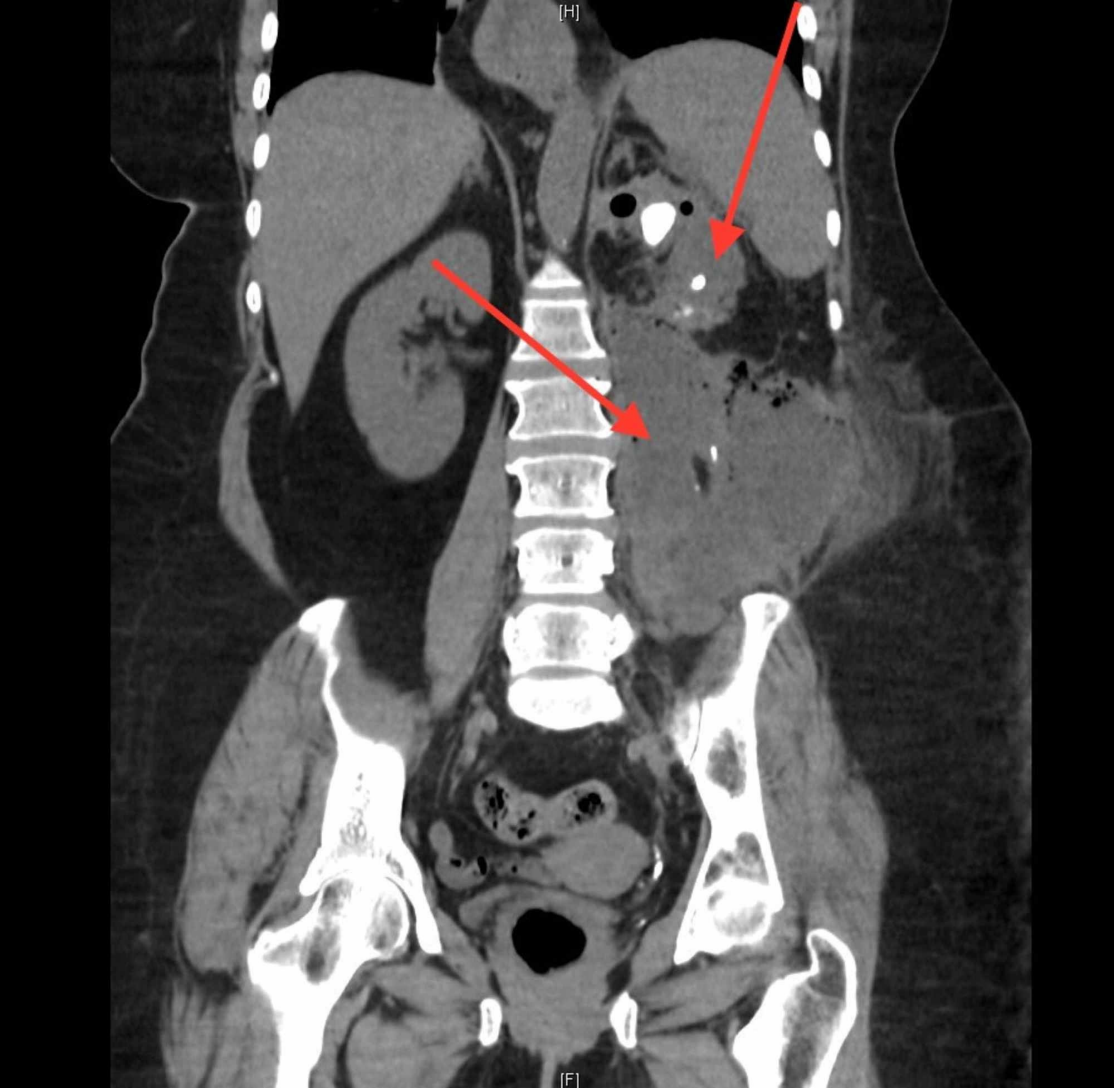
Non-contrast CT: Xanthogranulomatous emphysematous pyelonephritis w/large abscess The top arrow identifies nephrolithiasis and emphysematous pyelonephritis. The bottom arrow identifies the associated perinephric abscess with extension into the pelvic cavity.
